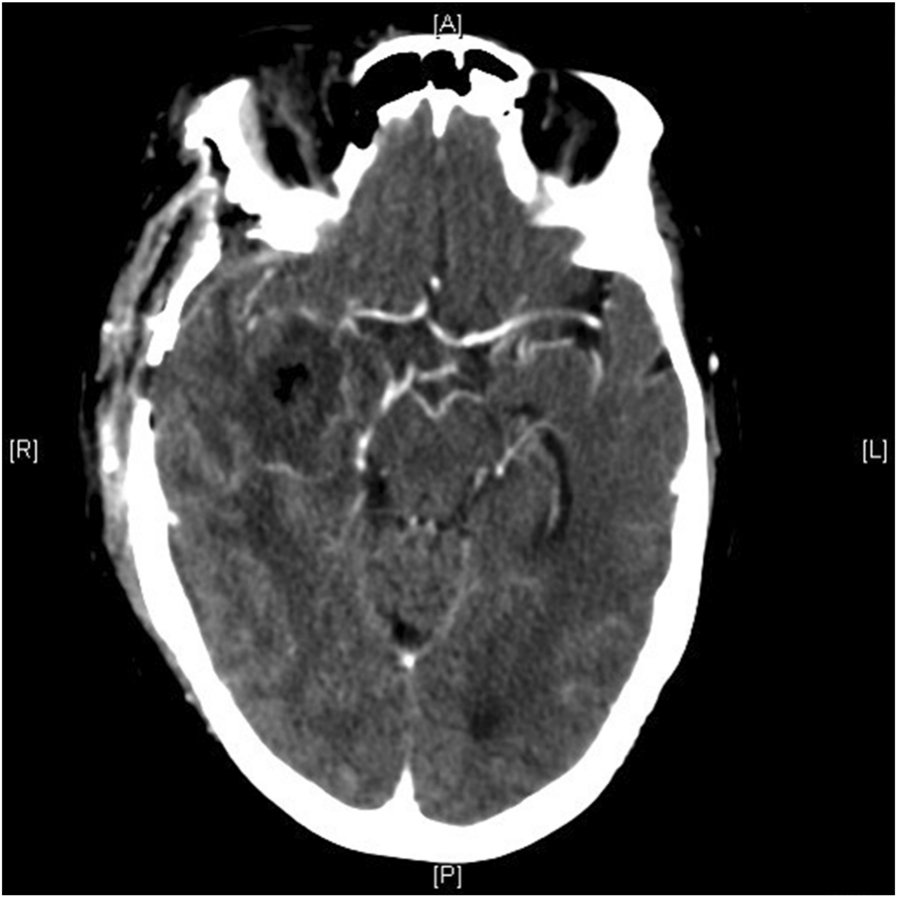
Computed tomography reveals a large cerebral abscess located at the operative site
